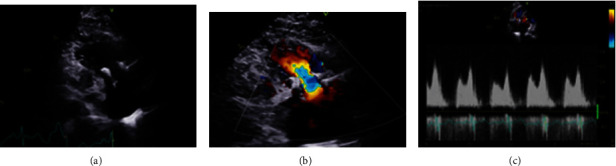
The transthoracic echocardiogram images during the 34-month follow-up period: TTE showed optimal valve position and well hemodynamic status (a, b). TTE with color flow showed a maximal velocity of 1.6 m/s (c).
